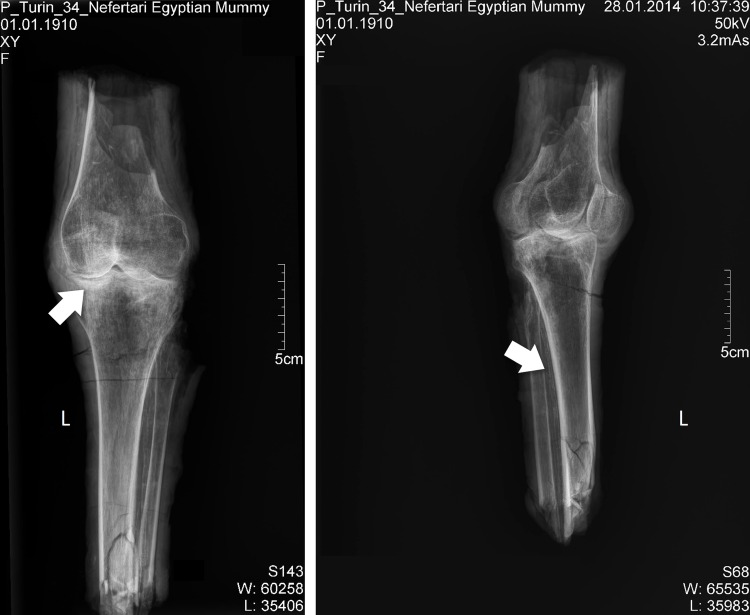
Left: X-ray left knee; the arrow marks the minimal signs of arthritis. Right: lateral view; the arrow points to the calcification of the arteriae tibiales.
